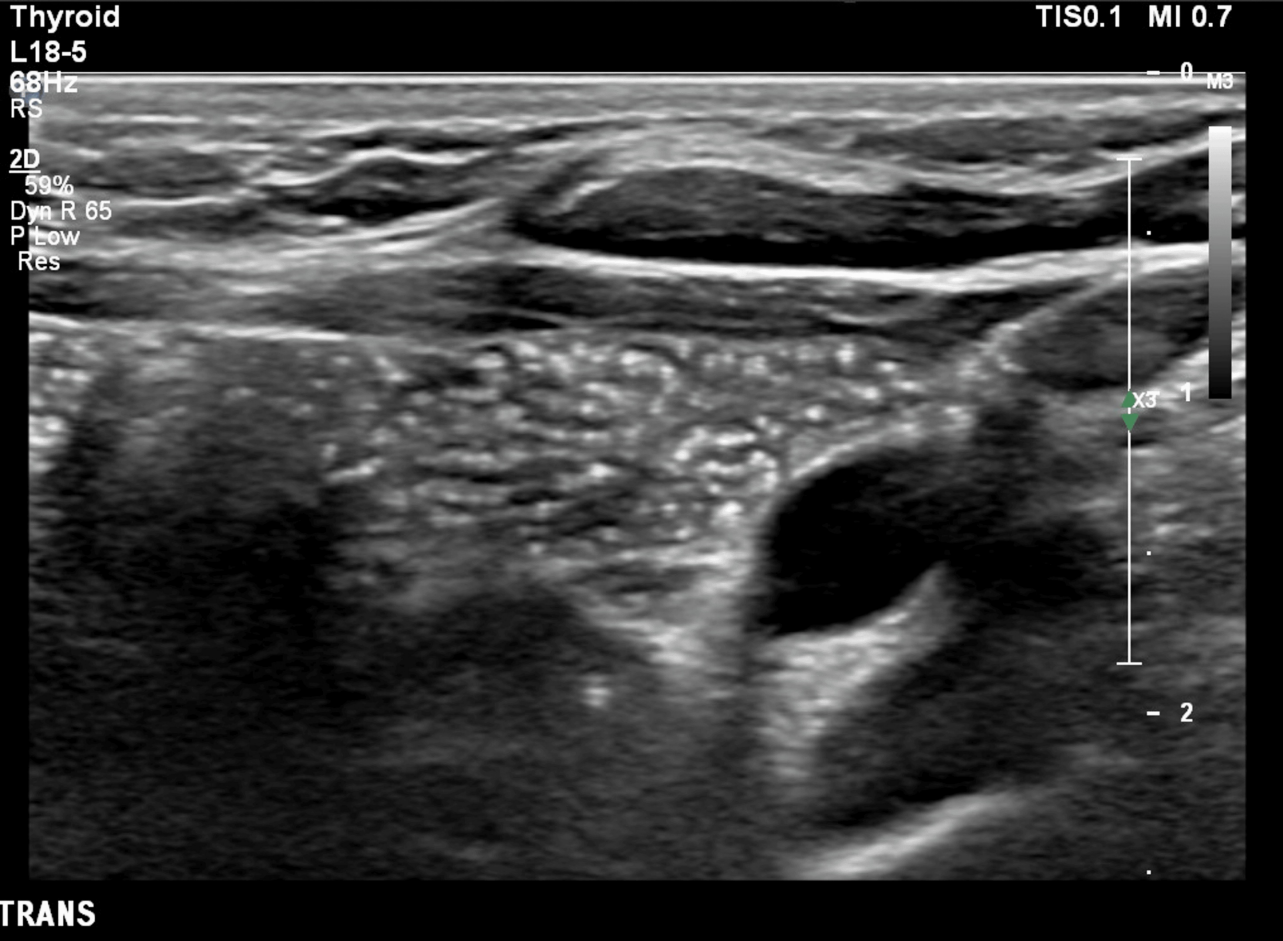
Transverse high-resolution ultrasound of the thymus demonstrating the characteristic starry-sky appearance due to multiple linear and punctate echogenic foci
